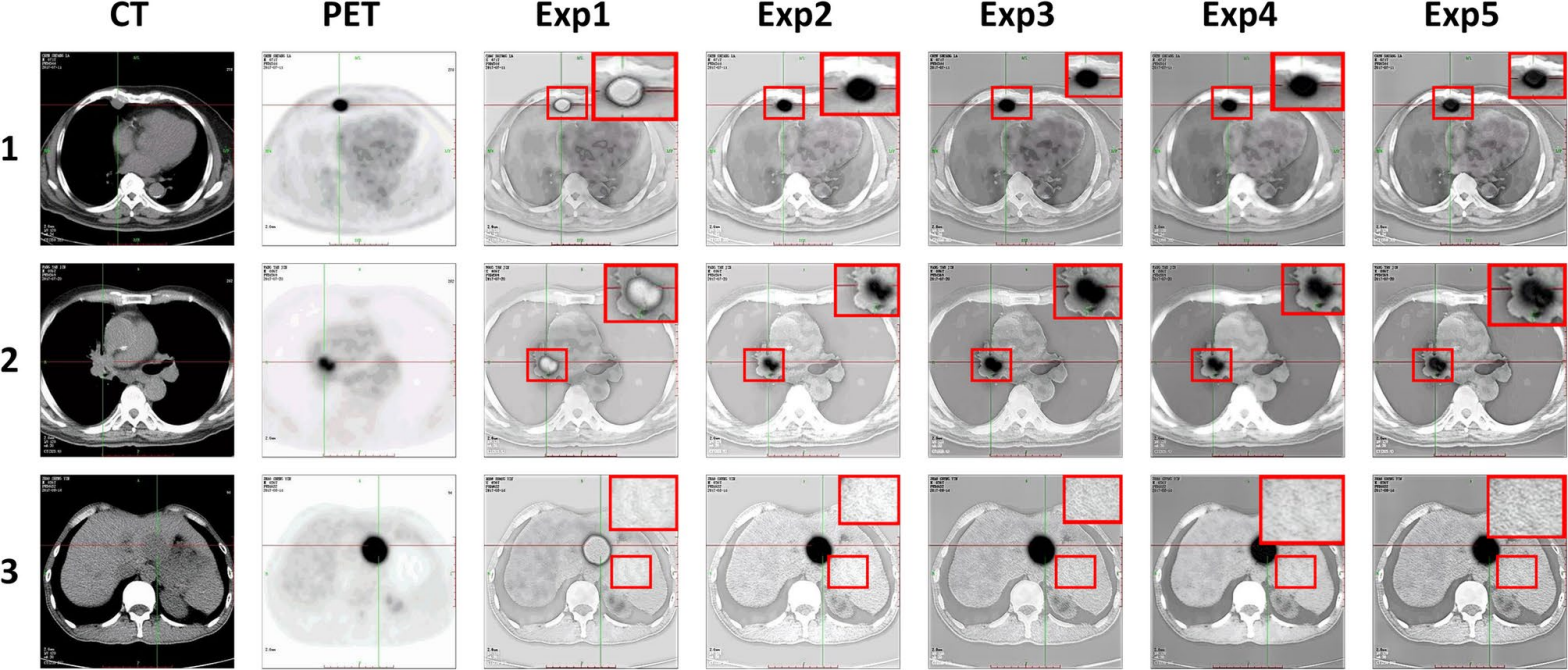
The ablation experiments fusion results of CT mediastinal window images and PET images.
